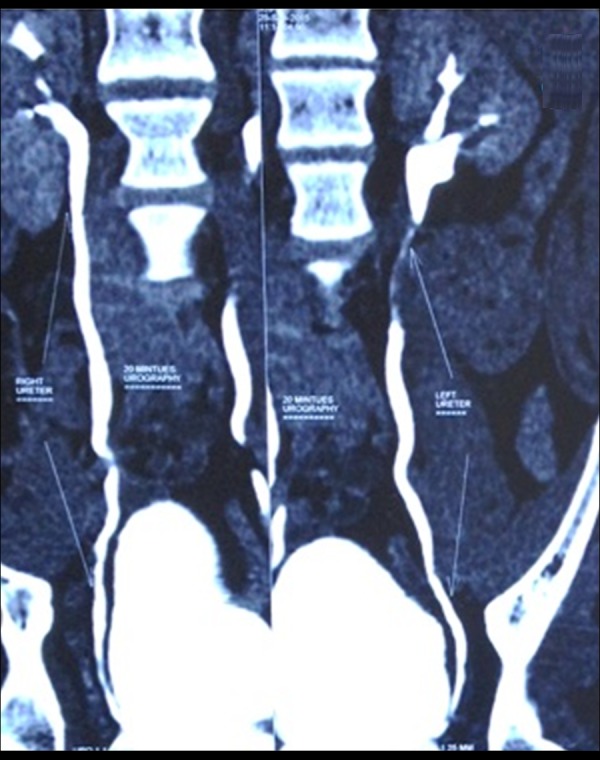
Coronal CT urography image showing filling defect in the left upper ureter; the right ureter appears unremarkable.
